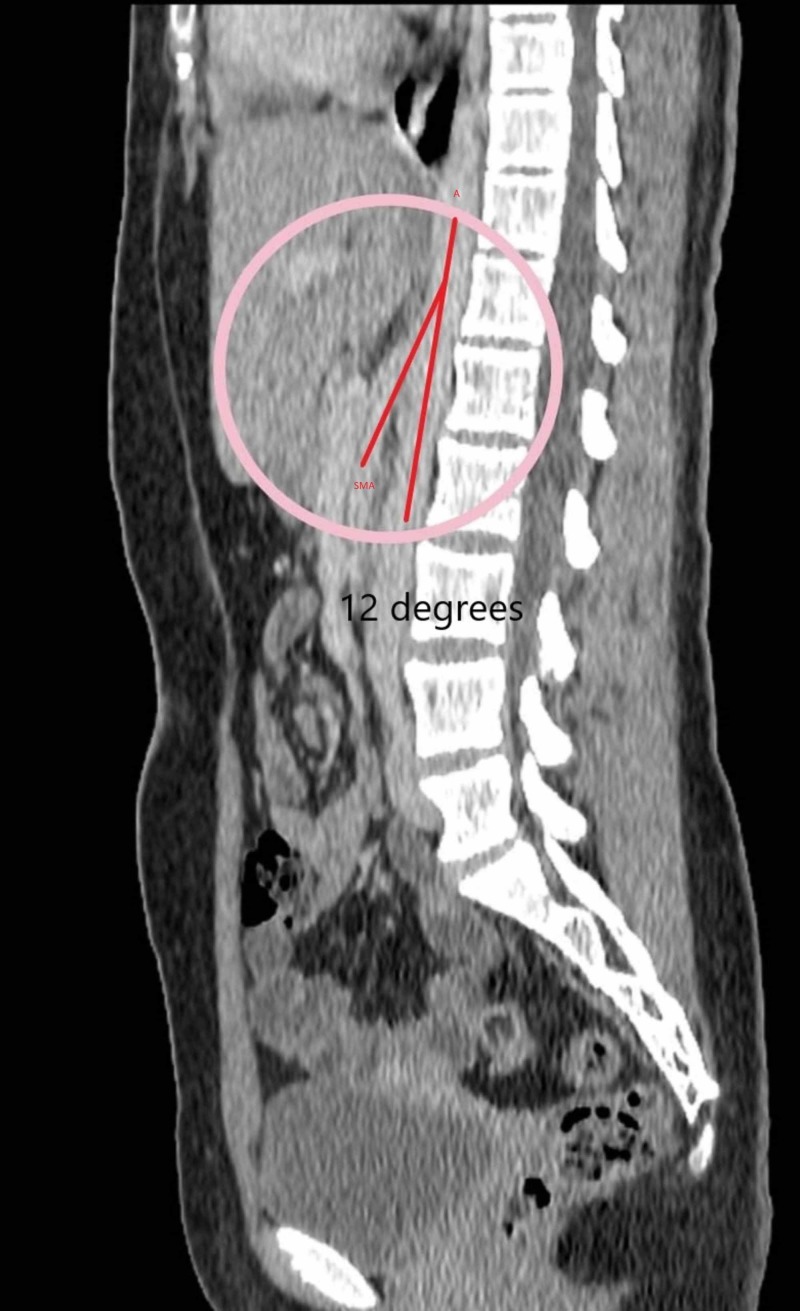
Computed tomography (CT) sagittal view showing aortomesenteric angle of 12 degrees by two red lines drawn over the aortic axis (A) and the superior mesenteric artery (SMA) at the origin (inscribed in a circle)
